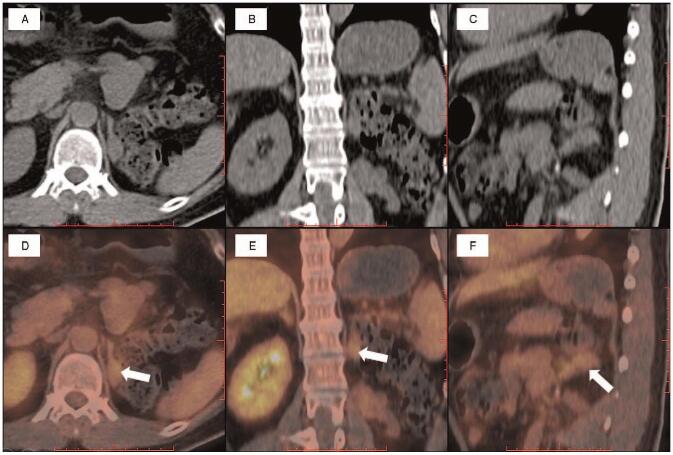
PET/CT scan showed high radio uptake mass on left adrenal gland (white arrows). (A&D) Axial PET/CT images: CT image and fusion image. (B&E) Coronal PET/CT images: CT image and fusion image. (C&F) Sagittal PET/CT images: CT image and fusion image.
